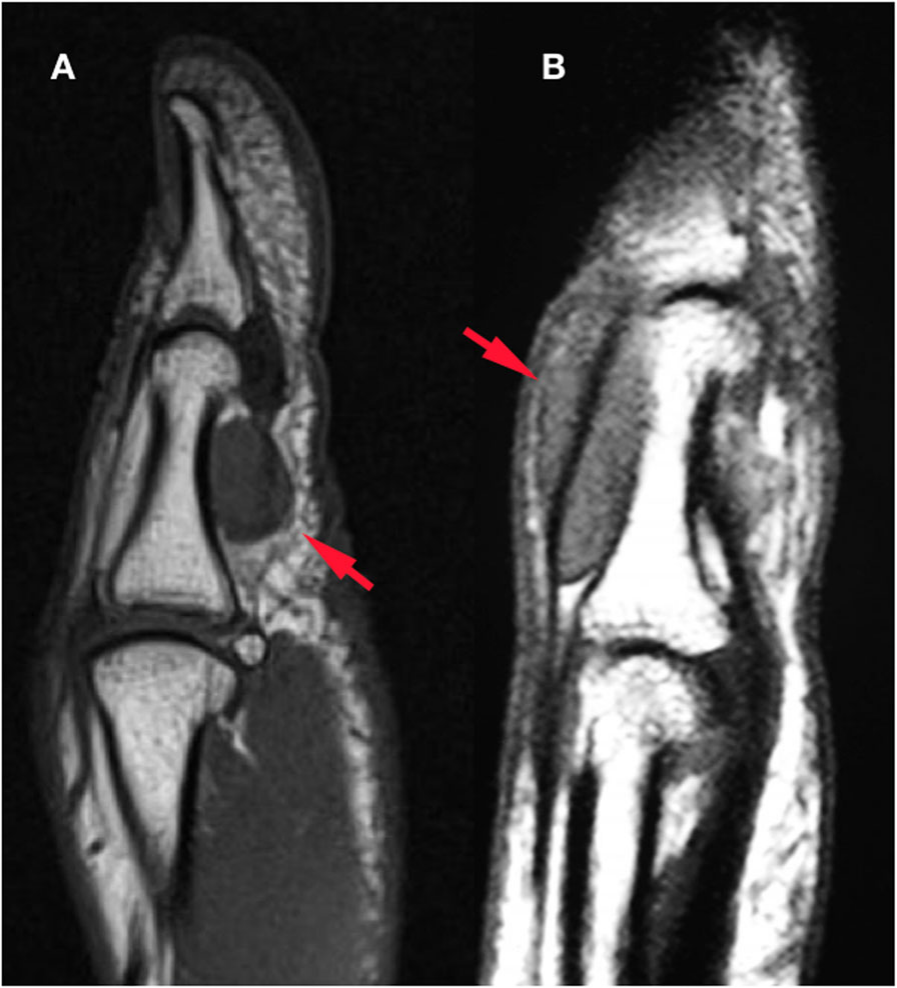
MRI clearly shows periosteal contact in GCTTS that was localized volarly (a) and dorsally (b)
